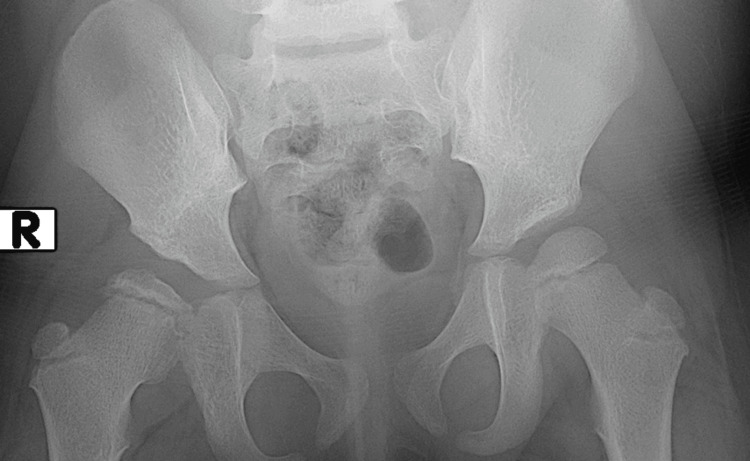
Most recent follow-up radiograph demonstrating restored Shenton-Menard line, deformity of the femoral head consistent with coxa plana, and increased acetabular index
